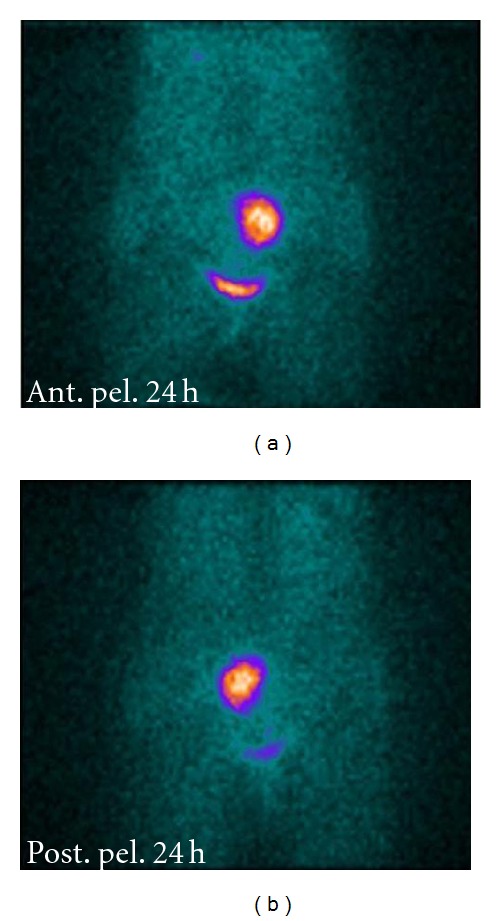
I-MIBG scintigraphy showing accumulation of radiopharmaceutical in the left paramedian site of the pelvis.
